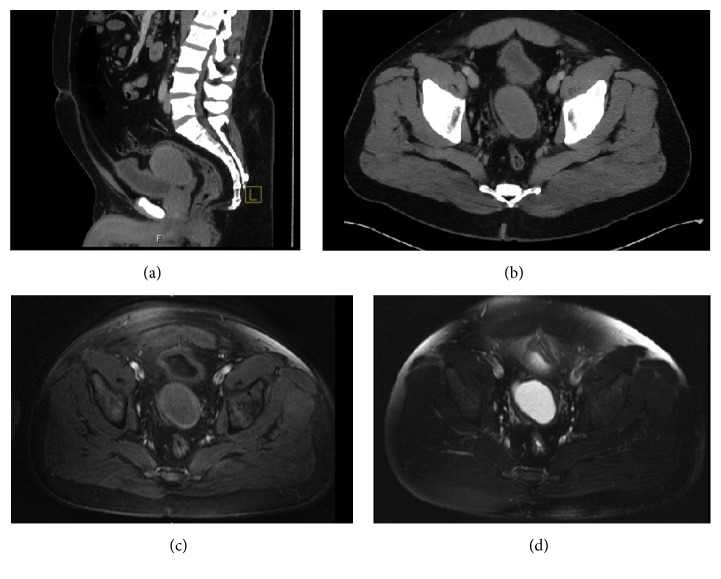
Computed tomography scan with contrast [(a) sagittal and (b) axial] displaying a complex midline cystic pelvic mass with thick walls found between the bladder and the rectum. Sagittal MRI of the pelvic mass with (c) T1-weighted imaging and (d) intensity on T2-weighted imaging.
